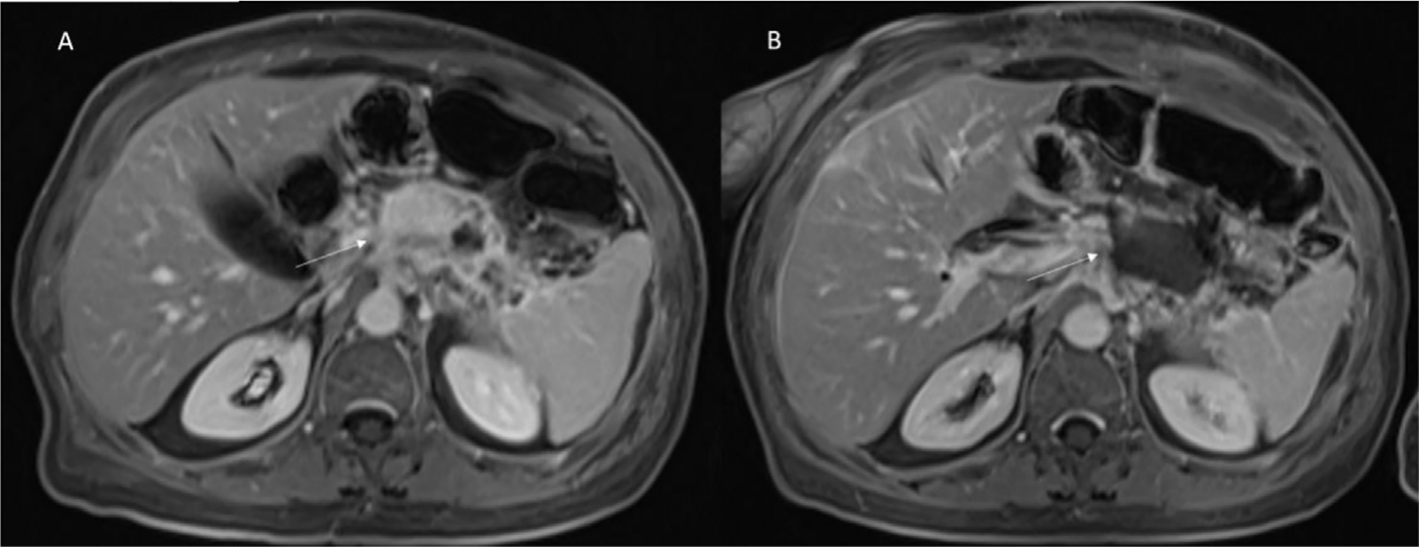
Patient 2 with Body-Tail Pancreatic Cancer. Morphological MRI assessment post-ECT treatment. In (A) (VIBE T1-W post-contrast sequence during portal phase in axial plane) pre-treatment evaluation of lesion (arrow). In (B) (VIBE T1-W post-contrast sequence during portal phase in axial plane) arrow shows ablated area. Qualitative assessment shows significant differences in SI in pre- and post-treatment sequences.
